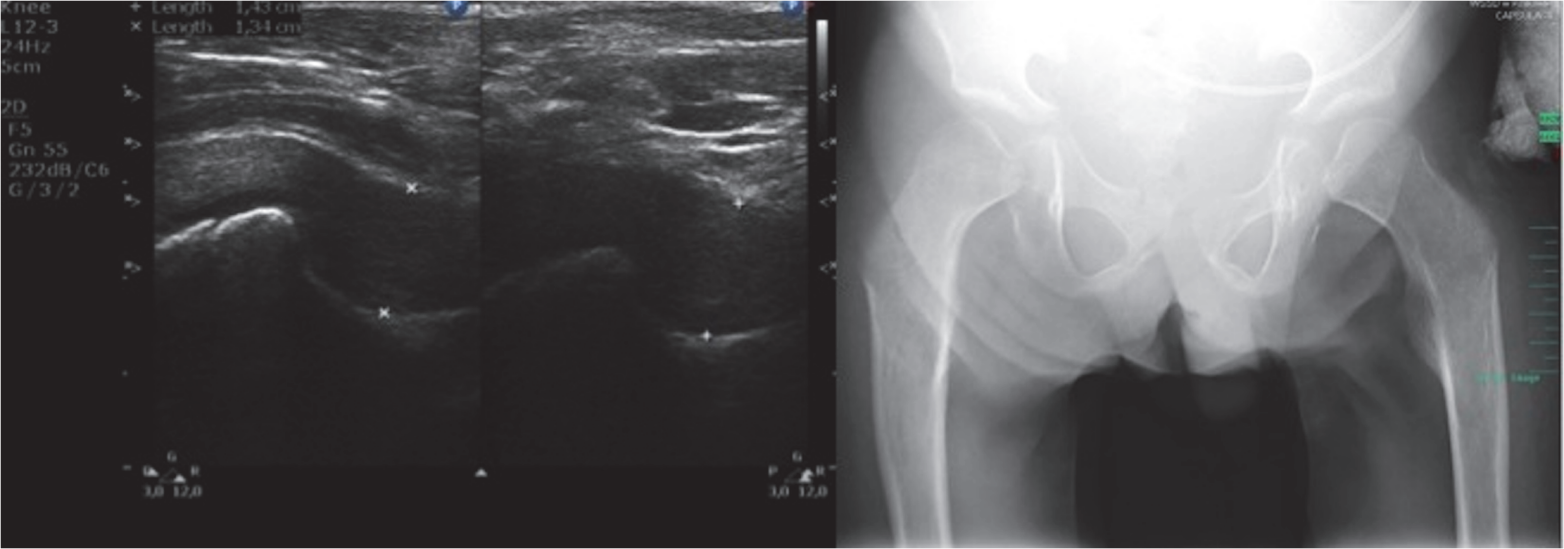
Ultrasound and X-ray images of hip joints. (Left) Transverse scan of hip joint a 12-year-old patient with a severe phenotype of MPS II. (Right) Radiograph of the pelvis of a 12-year-old patient with a severe phenotype of MPS II: dysostosis multiplex (irregular shape of the pelvis, hypoplastic hip acetabulum, dysplastic hips, osteonecrosis of the femoral heads with flattened acetabula, lopsided head of femur bones).
